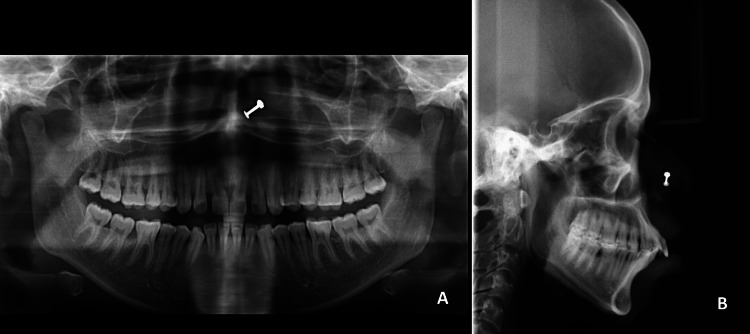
Pre-treatment radiographs. A: Orthopantomogram; B: Lateral cephalogram
